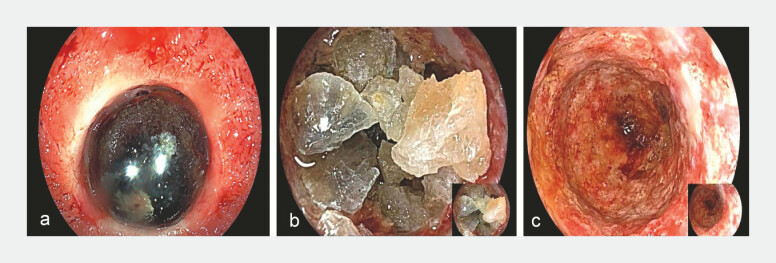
The combination of endoscopic ultrasound-guided gallbladder drainage and electrohydraulic lithotripsy.
